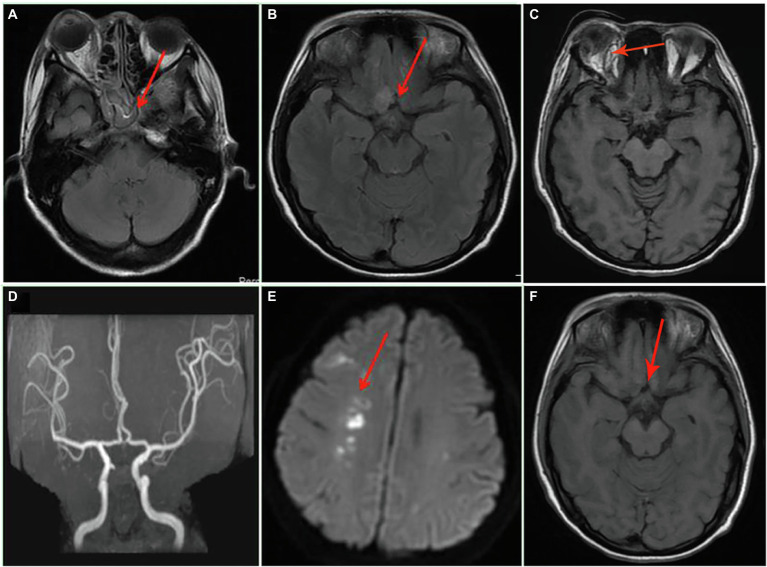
Axial MRI and magnetic resonance angiography of the brain on admission and after admission. (A) Inflammation of the sphenoid sinus and sinuses; (B) the frontal lobe lesion; (C) orbital MRI; (D) the normal magnetic resonance angiography; (E) the new cerebral infarction at the right basal ganglia 17 days after admission; and (F) the presentation of frontal lobe lesion after 67 days of treatment.
